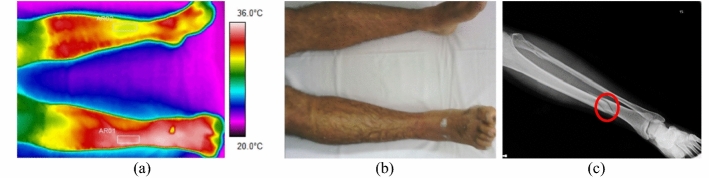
Fracture of the right tibia: a infrared image of the patient's lower limbs with the delimitation of the ROIs; b photograph for comparative analysis; c Radiographic image of the fracture
